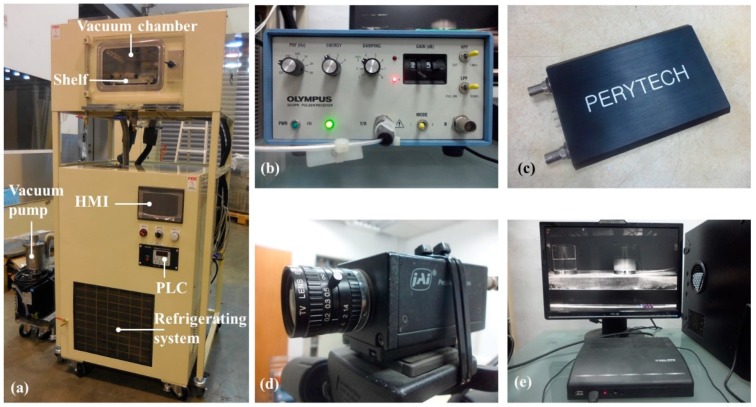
Photographs of (a) the split-type freeze-drying machine with vacuum chamber, controller unit, refrigeration unit and vacuum pump, (b) the ultrasonic pulse/receive unit, (c) a PC-based digital oscilloscope, (d) Charge-coupled device (CCD) camera, (e) digital video recorder (DVR) and PC.
